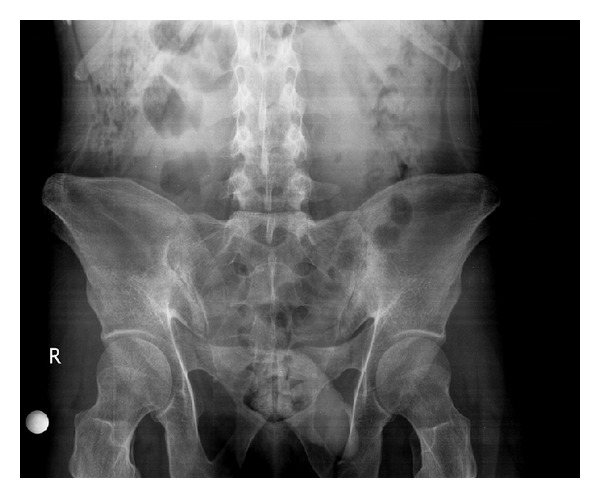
Direct radiography of the sacroiliac joints showing bilateral sacroiliitis.
